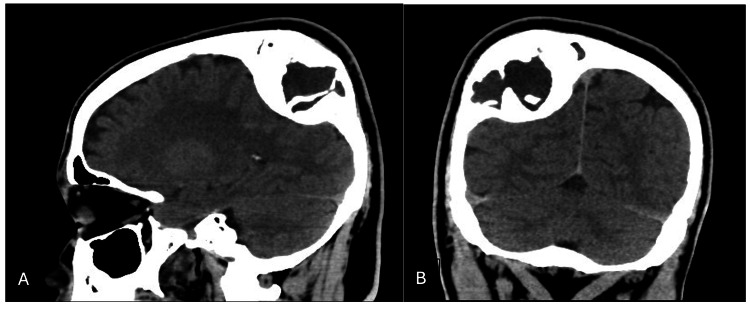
Preoperative non-contrast computed tomography scan. Both sagittal (A) and coronal (B) planes show hypertrophied right parietal sclerotic bone lesions, compatible with fibrous dysplasia.
